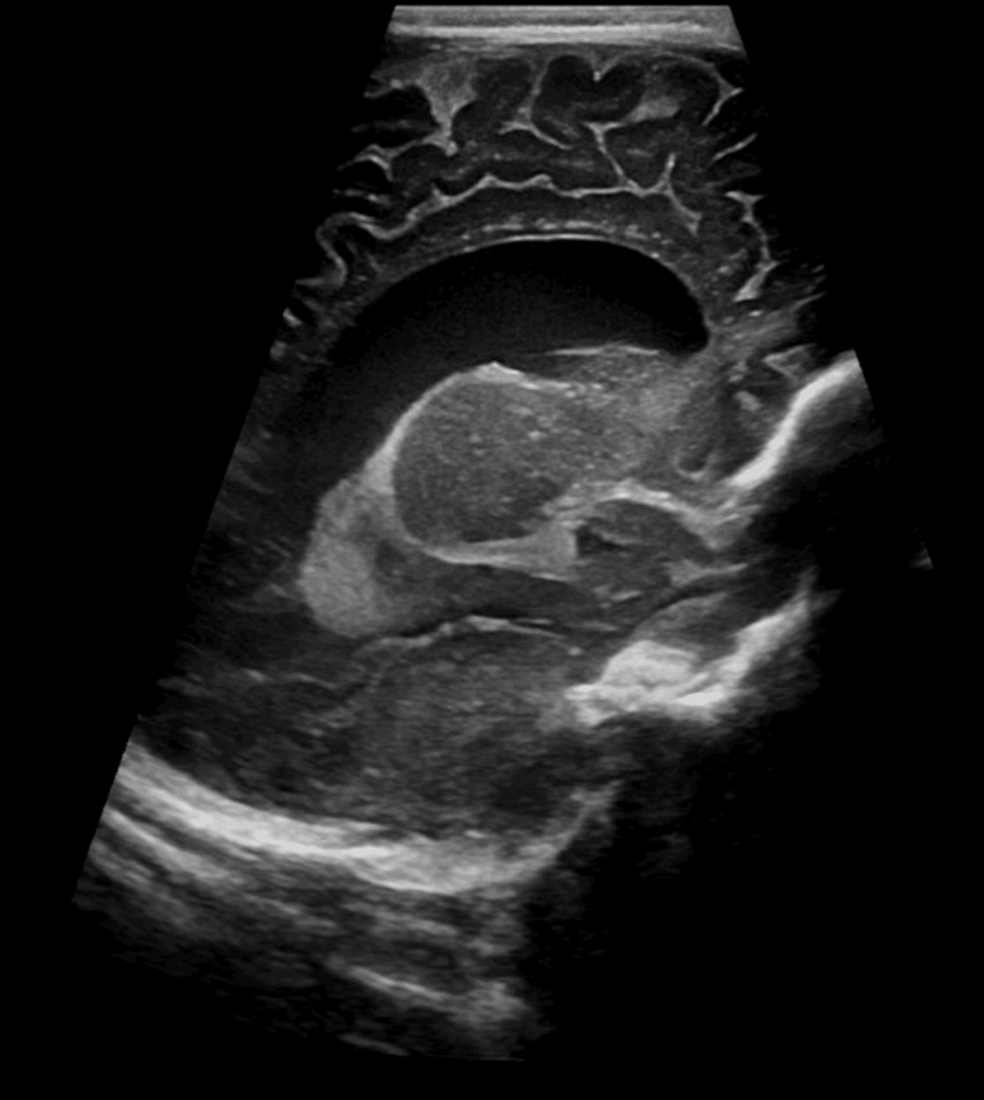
Ultrasound of the head depicting ventriculomegaly.
